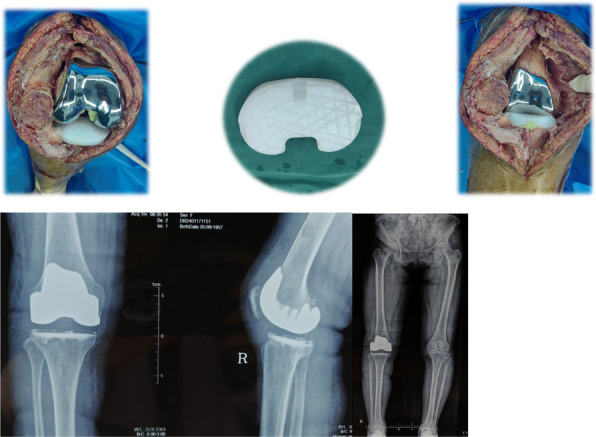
The process of performing the prosthetic spacer and postoperative X-ray
